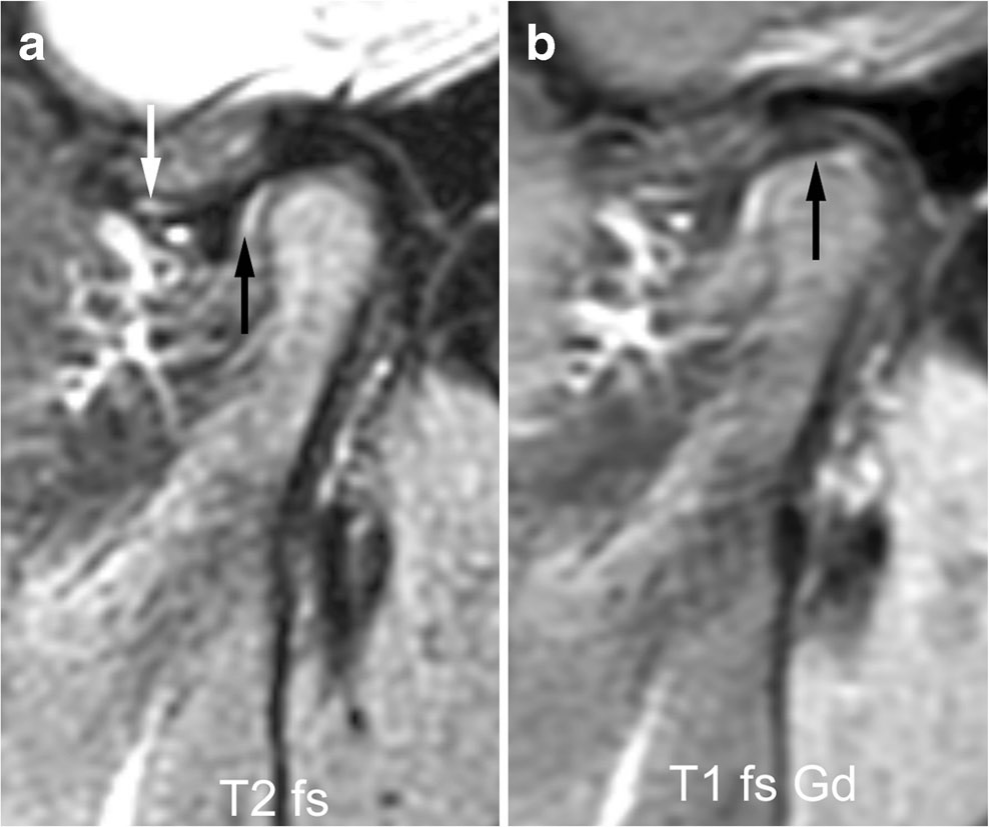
No synovial thickening (grade 0) but mild increase of joint enhancement (grade 1) on sagittal oblique images in a 6-year-old boy. a Fat saturated T2-weighted image (TR/TE 5,400/77 ms) shows normal amount of joint fluid in upper and lower anterior recesses (arrows). b Postcontrast T1-weighted fat-saturated image (TR/TE 670/10 ms) shows high signal intensity, isointense to that of veins, in both joint compartments. At the midportion of the lower joint compartment (arrow) this exceeds the extent of high signal intensity seen in (a). Note mild bone marrow oedema and enhancement of the normally shaped mandibular condyle. T1 fs Gd contrast-enhanced fat saturated T1-weighted, T2 fs fat saturated T2-weighted
